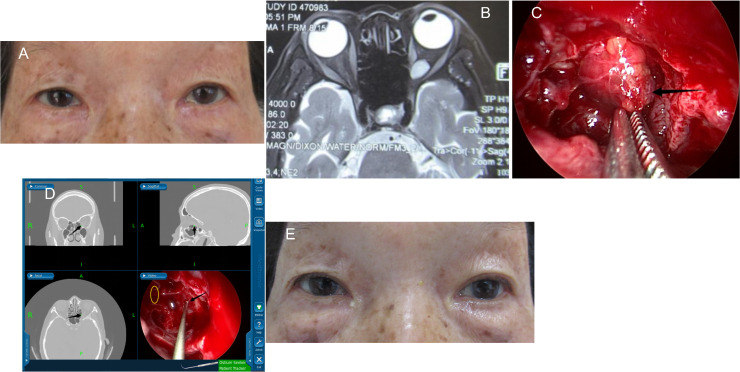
Patient P2 - endoscopic transnasal resection of left orbital apex cavernous hemangioma. (A) Preoperative facial appearance. (B) Axial MRI showing the left orbital apex cavernous hemangioma. (C) Endoscopic transnasal surgical removal of the tumor (black arrow). (D) Intraoperative navigation localization of the tumor (black arrow), skull base (yellow circle). (E) Postoperative facial appearance.
